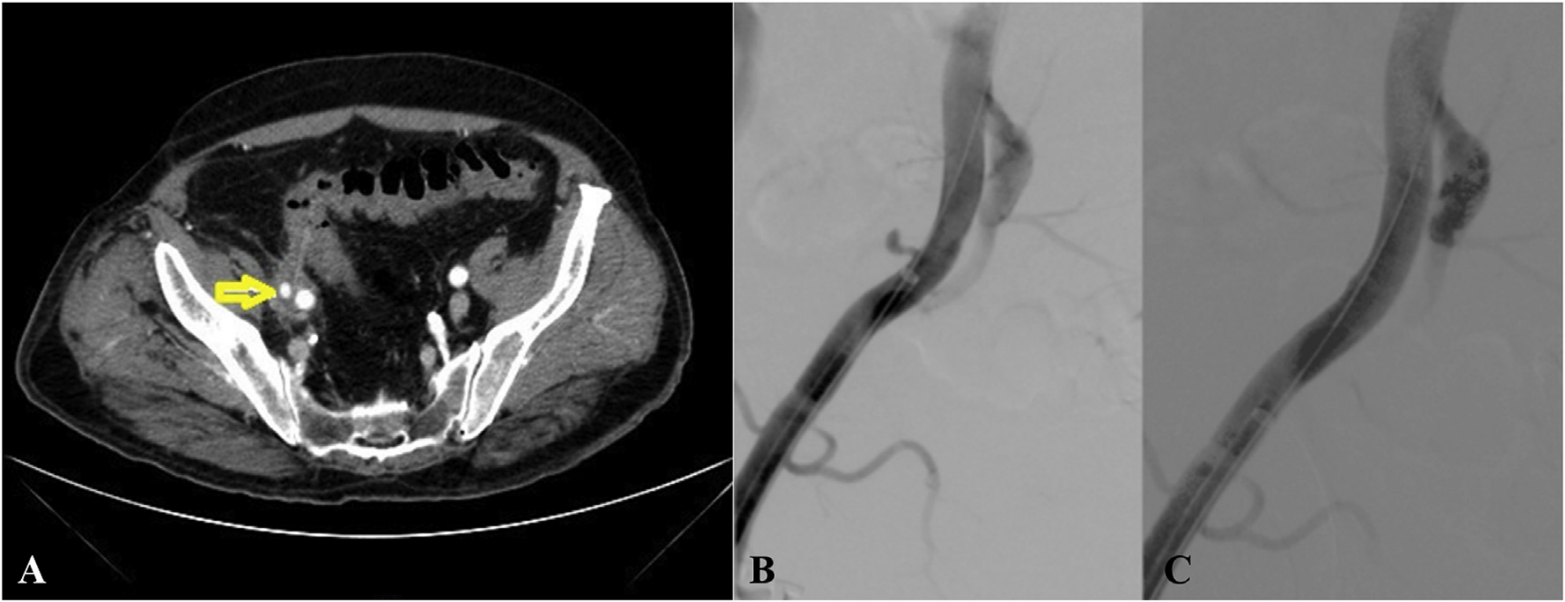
A: CT Angio-scan: a roundish formation of about 1 cm with intense arterial enhancement, referable in first hypothesis to a pseudoaneurysm of the right external iliac artery. B: Intraoperative angiography showing external iliac pseudoaneurysm. C: Completing angiography with correct stent positioning and exclusion of the pseudoaneurysm.
